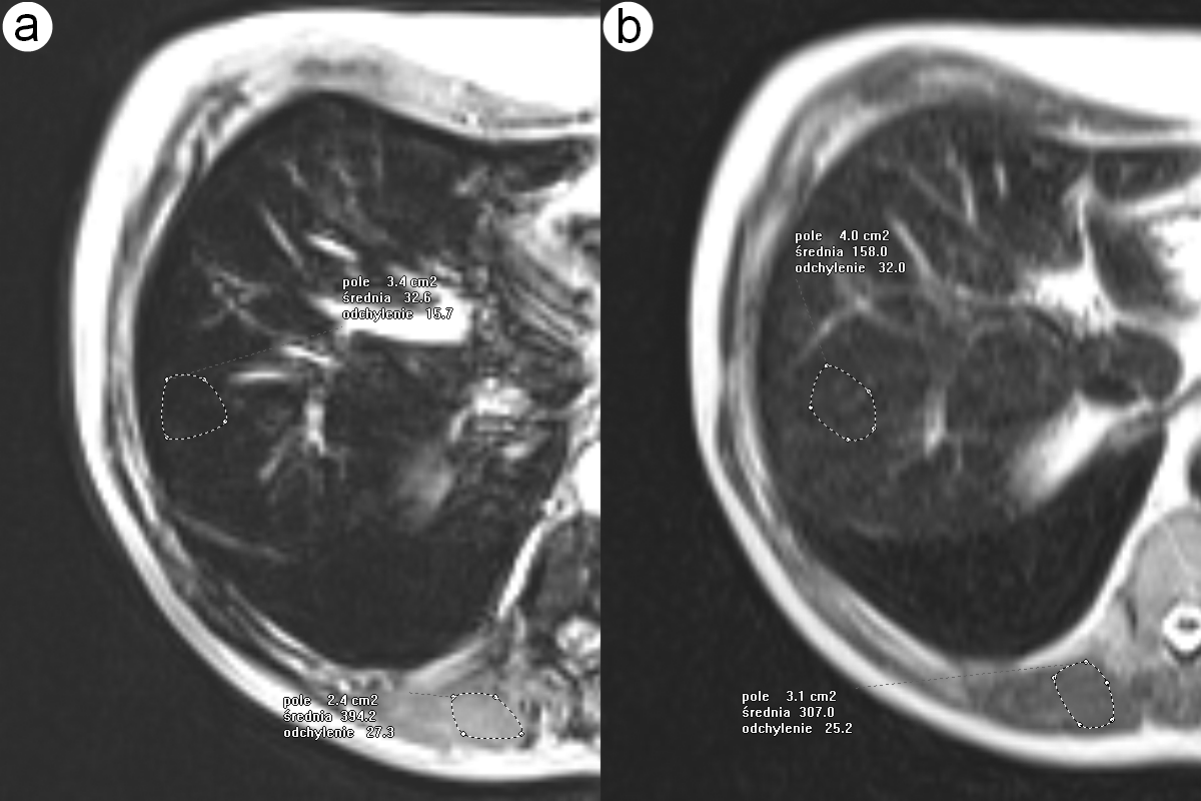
45-year-old man with cirrhosis and hemochromatosis confirmed by the biopsy and genetic examination. Axial MR T2-weighted images performed on gradient echo (fig.3a) and Express (fig.3b) sequence show typical findings for hepatic iron overload. The signal intensity of liver (accordingly 33 and 158) is higher than that of paraspinal muscles (accordingly 394 and 307).
